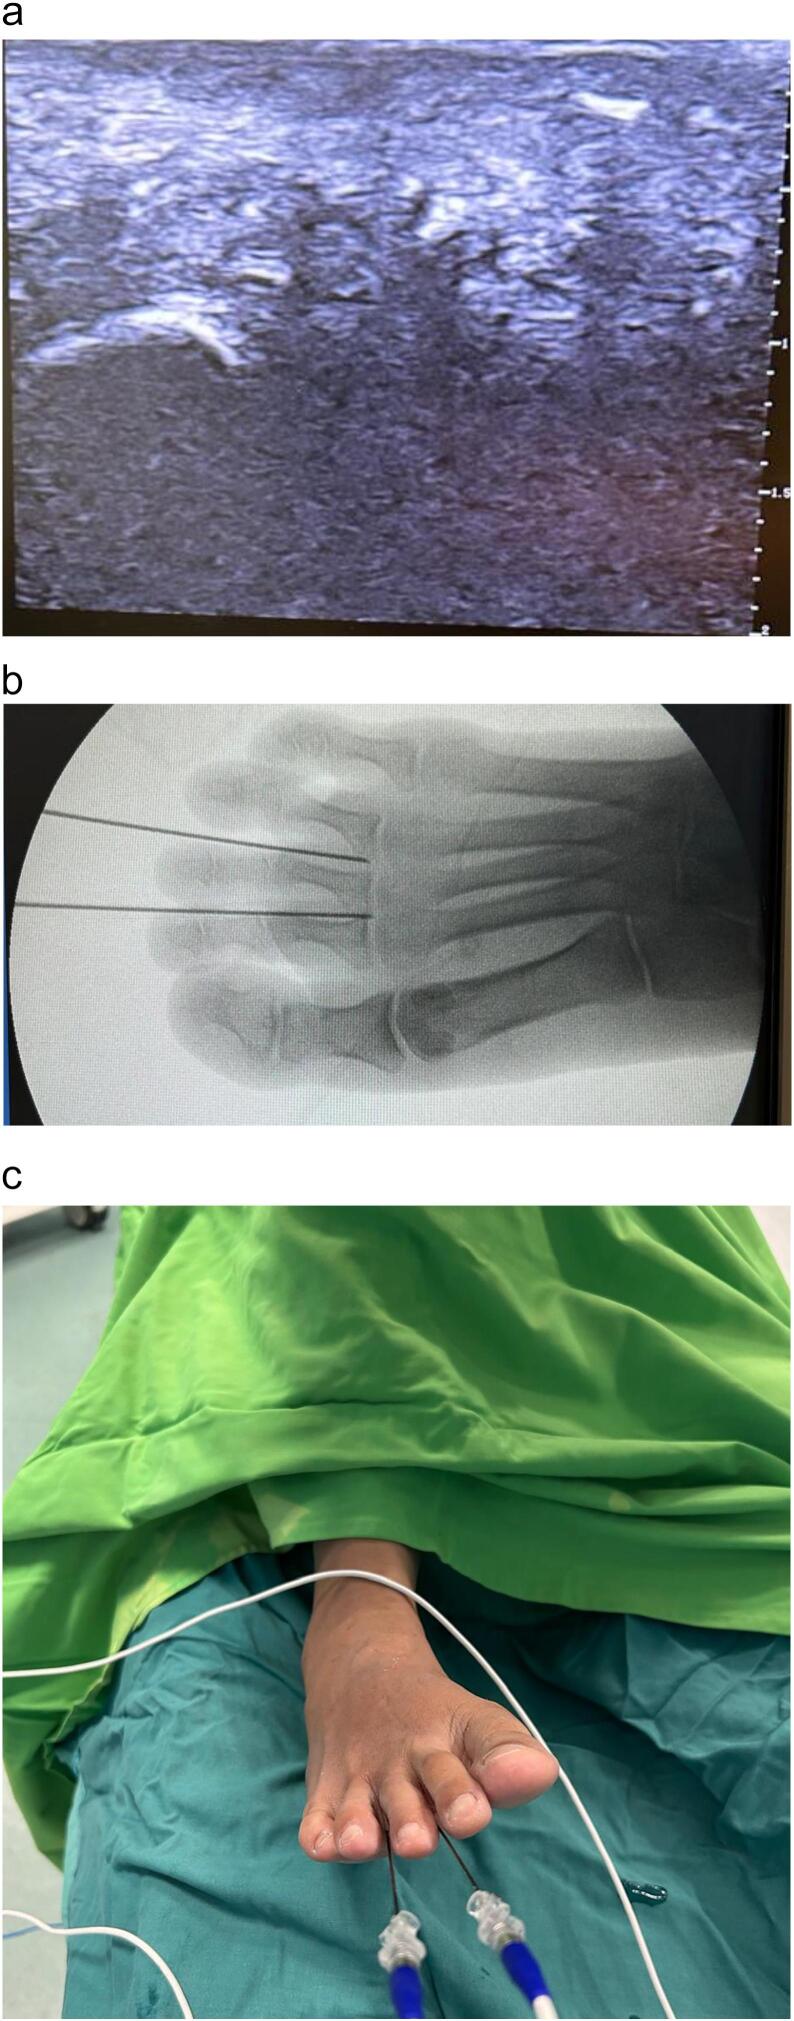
A linear ultrasound shows a hypoechoic Morton's Neuroma (a). A cannula position at Morton's Neuroma lesion around the distal part of two-thirds of the intermetatarsal was confirmed by fluoroscopy (b). Two RF cannulas were inserted at the foot's 2nd and 3rd web space for transmitting PRF (c).
